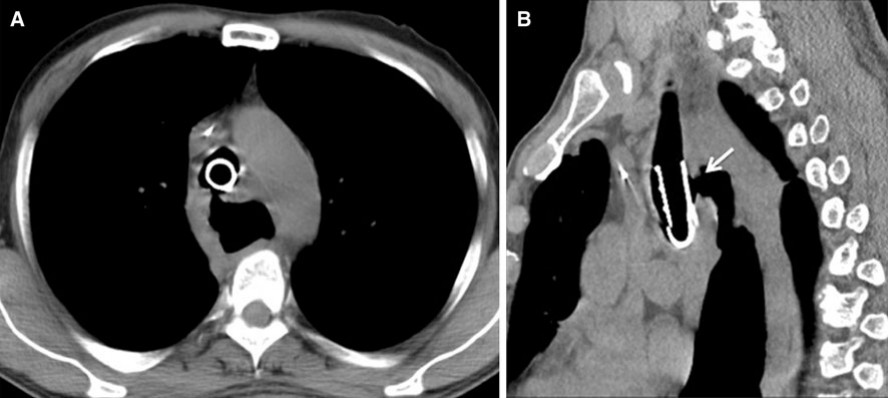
Preoperative chest computed tomography (CT) shows a tracheoesophageal fistula. There is a space around the stent because of chemoradiotherapy (a) and a tracheoesophageal fistula at the upper thoracic esophagus (b). The *arrow* shows a tracheoesophageal fistula
